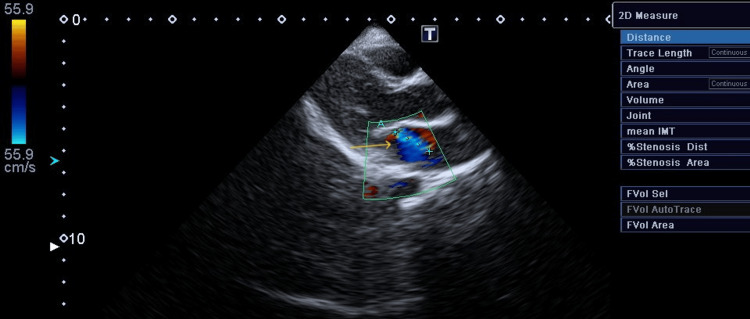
Mitral regurgitation on follow-up examination Echocardiography follow-up demonstrating mitral regurgitation measuring 15 mm in diameter with a velocity of 2.0 m/s (arrow).
